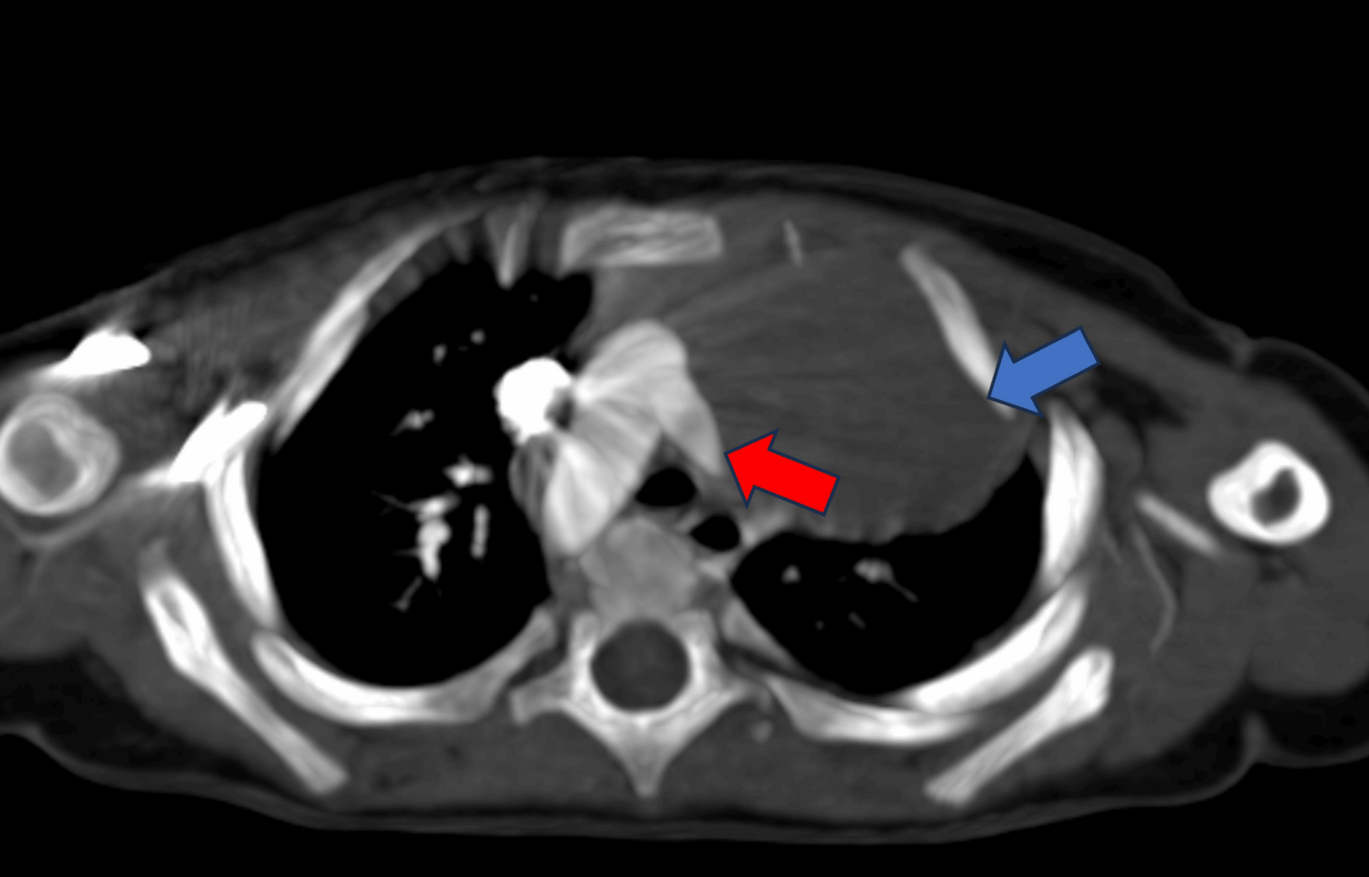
Computed tomography pulmonary angiography revealed an absent left pulmonary artery (red arrow) and left lung hypoplasia (blue arrow)
